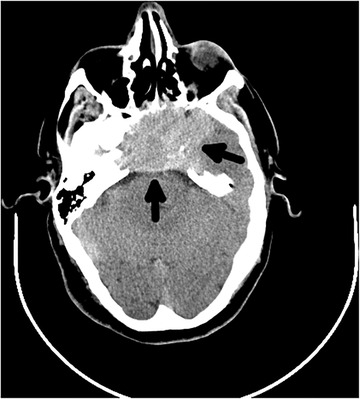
Axial plane of brain CT with a massive infiltrative lesion with 63 mm
